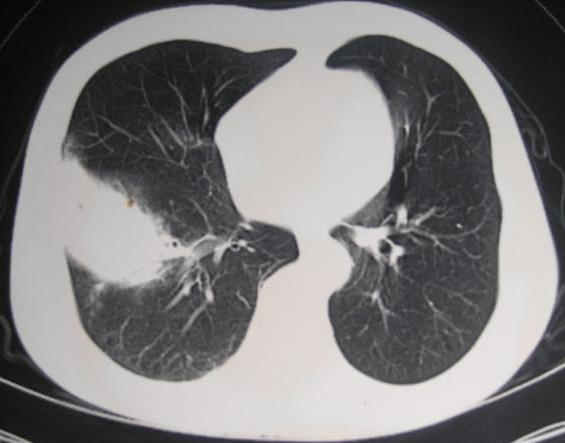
Computed tomography scan (GE Medical System. lightspeed 16) of the thorax showing features of lobar pneumonia. In this image right lung lower lobe soft tissue density shadow can be seen.
